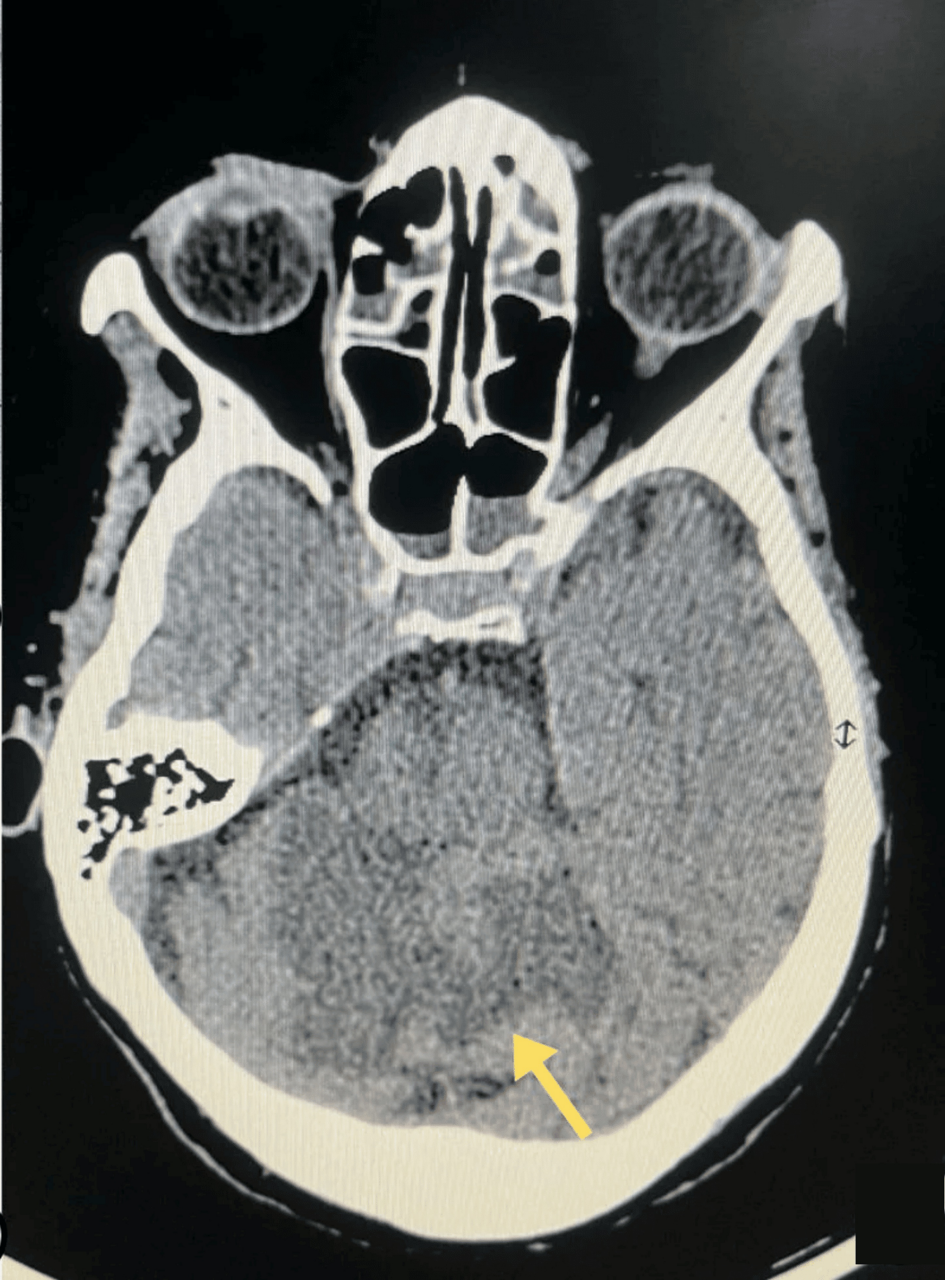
Axial non-contrast CT brain demonstrating acute cerebellar infarction (yellow arrow) without intracranial hemorrhage
